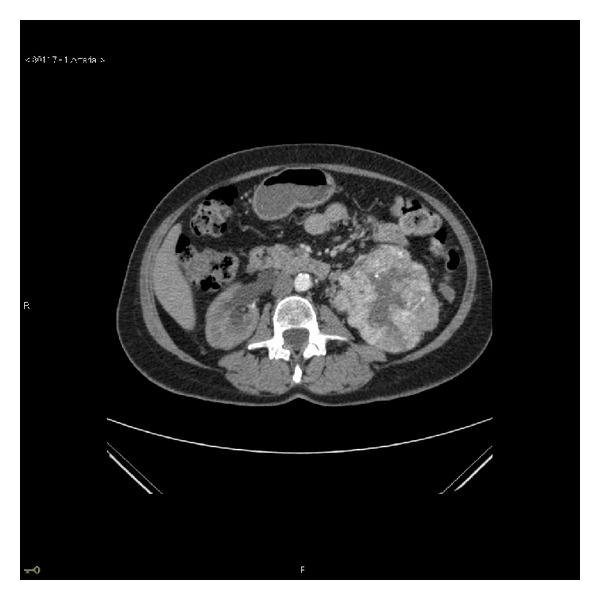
Axial arterial phase staging CT scan again demonstrating a 96 mm left renal mass.
